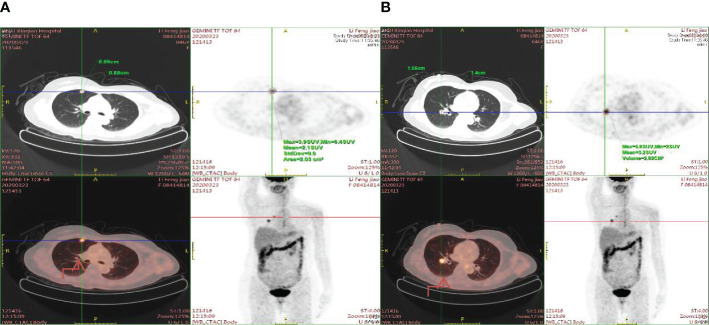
Enhanced radioactive uptake and a peak standardized uptake value (SUVmax) of 5.8 are observed in a larger nodule (1.06cm x 1.4cm) situated near the oblique fissure of the right lower lobe. (A) The nodule is situated in the right upper lung near the pleura. (B) The nodule is situated near the oblique fissure of the right lower lobe.
